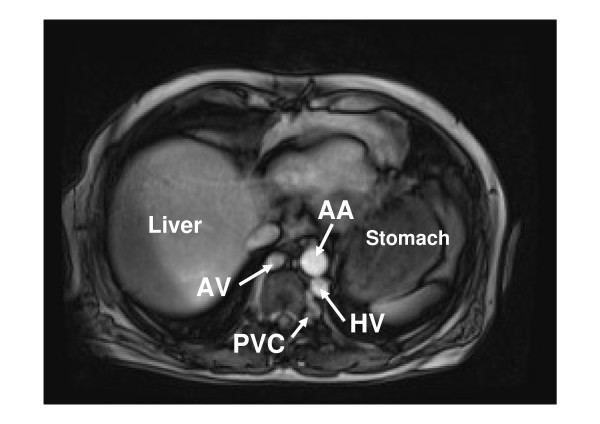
MRI demonstrating the hemiazygos vein (HV) and the azygos vein (AV) enlarged to compensate this anomaly. They collect the blood return from dilated paravertebral venous collaterals (PVC).
